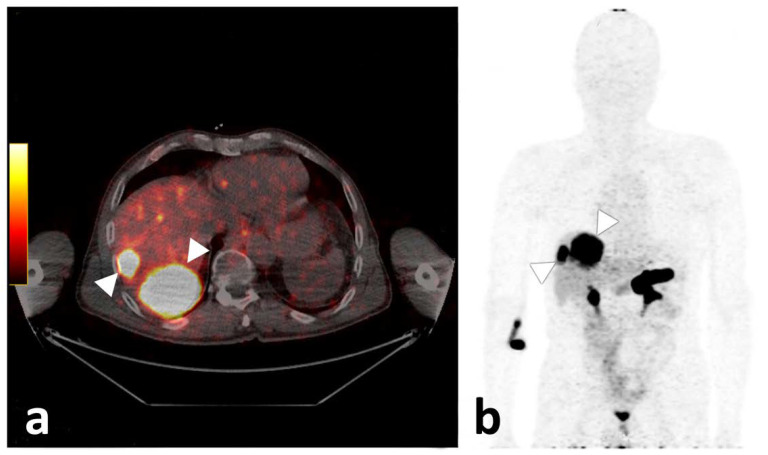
Axial fused PET/CT image of participant 3, demonstrating two liver metastases with high tracer uptake (a). On the (b), the corresponding maximum intensity projection is given, also demonstrating strong physiologic pancreatic as well as rectal and diffuse small bowel tracer accumulation.
